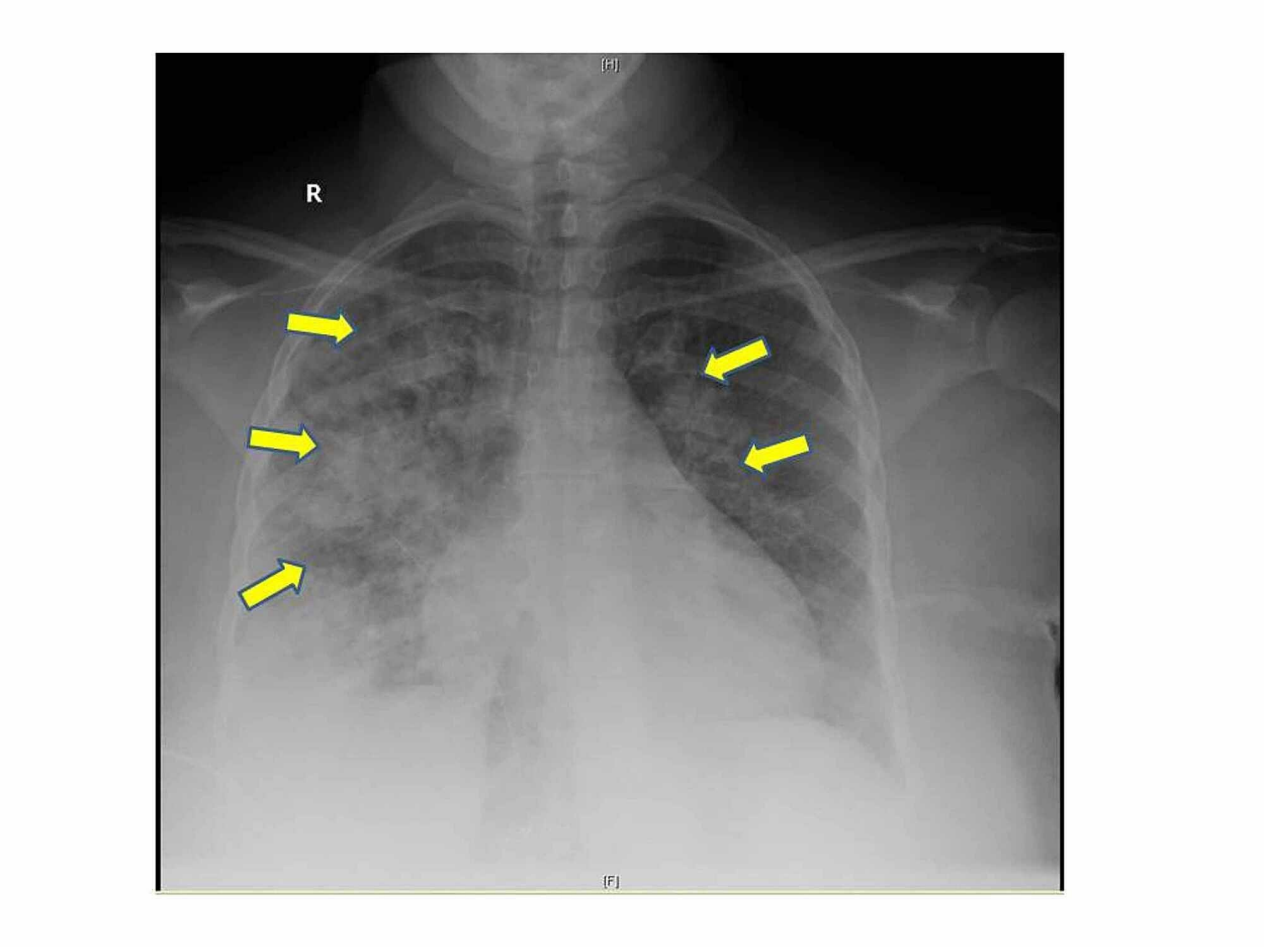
Chest X-ray of the patient showing diffuse bilateral infiltrates.
